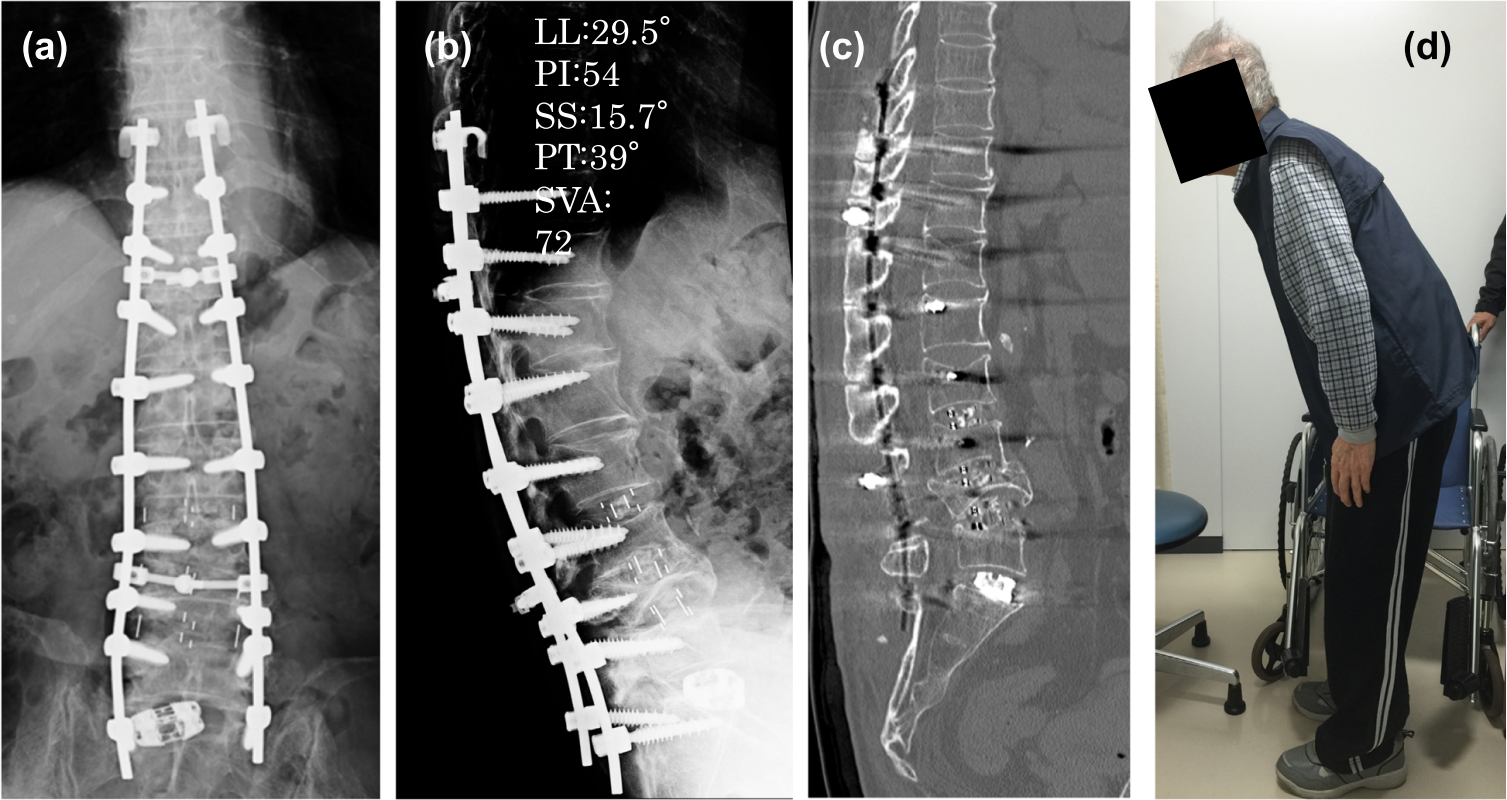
Postsurgical images. (a) Antero-posterior view. (b) Lateral view. (c) Computed tomography scan lateral image. (d) Maximum extension position. The patient can stand alone gazing straight forward.
